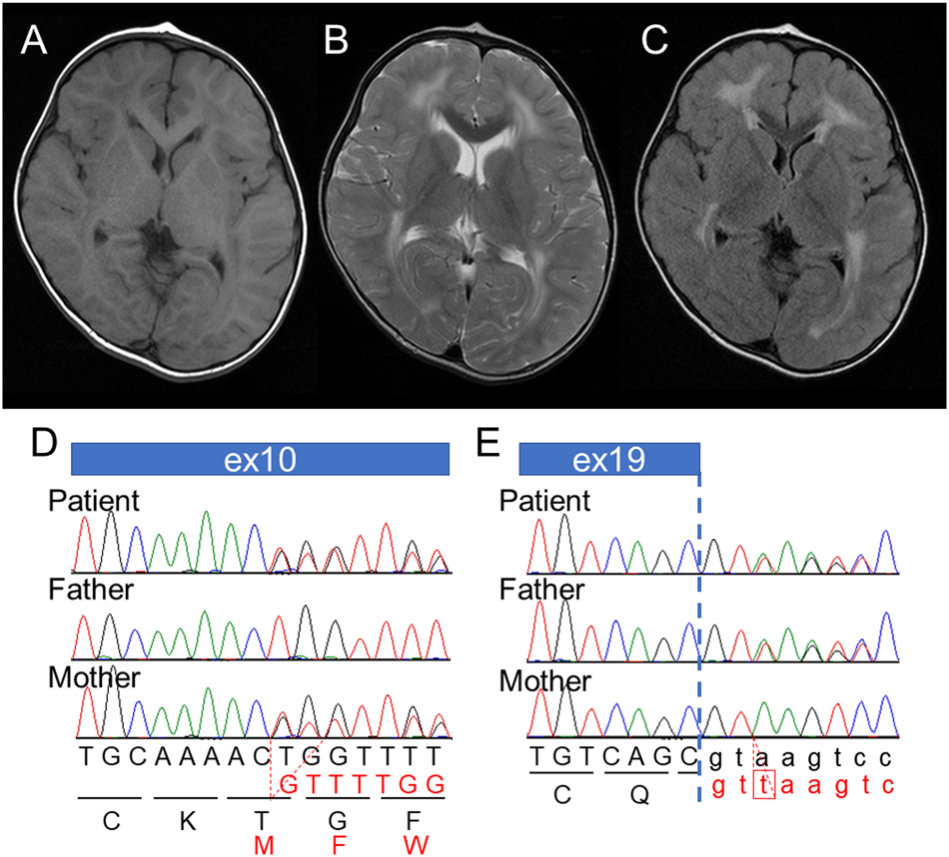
Patient information. a–c Brain MRI findings. T1 (a) and T2 (b) weighted axial images and a T2-flair axial image (c). T2- and T2-flair images show high signal intensity in the white matter (b, c). d, e Electropherograms of Sanger sequencing results. A 2-bp deletion is shown in the patient and his mother (d). 1 bp (t) is duplicated in the splicing donor site in the patient and his father (e).
